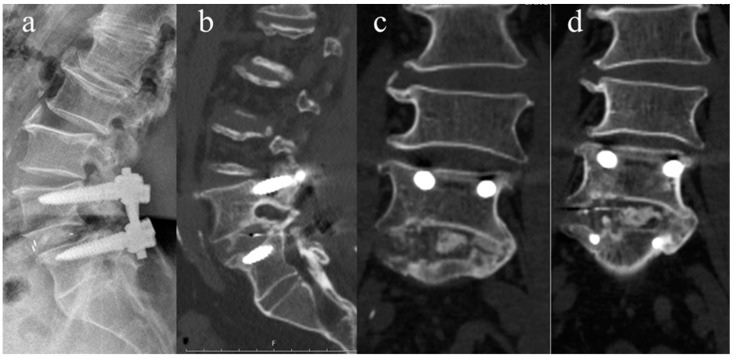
Representative plain X-ray and CT slices of a 72-year-old man who underwent 1 level TLIF. (a) Plain X-ray at 52 weeks after surgery, Grade I according to Bridwell classification (fused with remodeling and trabeculae present); (b–d) Grade II, which refers to partial fusion where the allografts cortex joins with the endplate in CT sagittal and coronal view. Abbreviations: CT—computed tomography.
